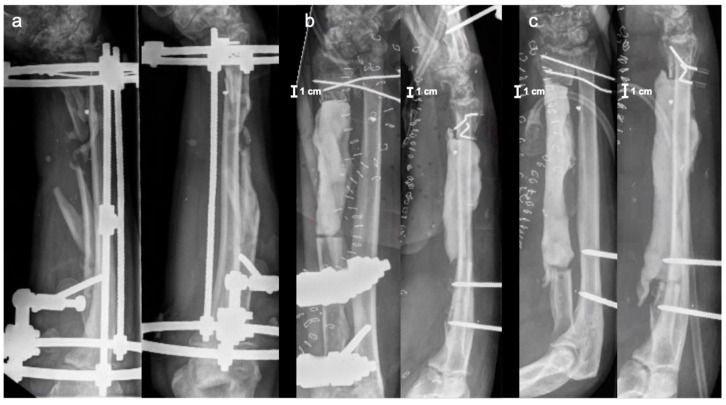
X-ray images (AP and lateral view) of the right forearm: (a) at the time of admission; (b) after 1st operation; and (c) before bone reconstructive surgery.
